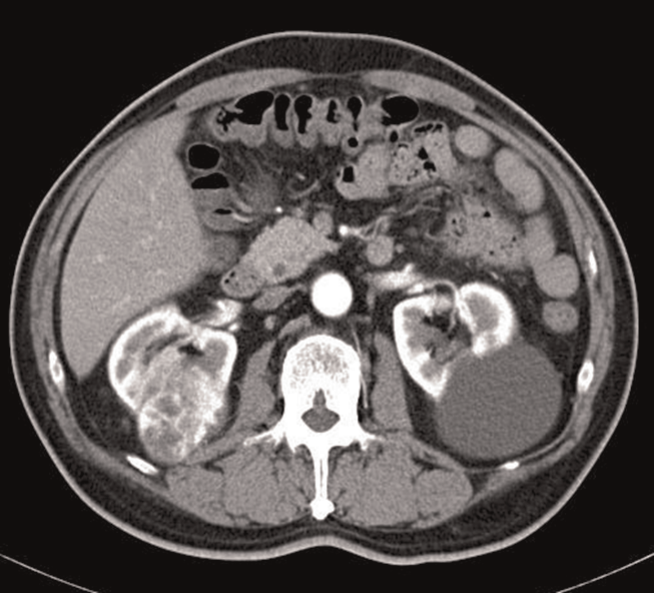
Abdominal CT before radical nephrectomy. Abdominal CT showed right hypervascular renal mass compatible with RCC.
